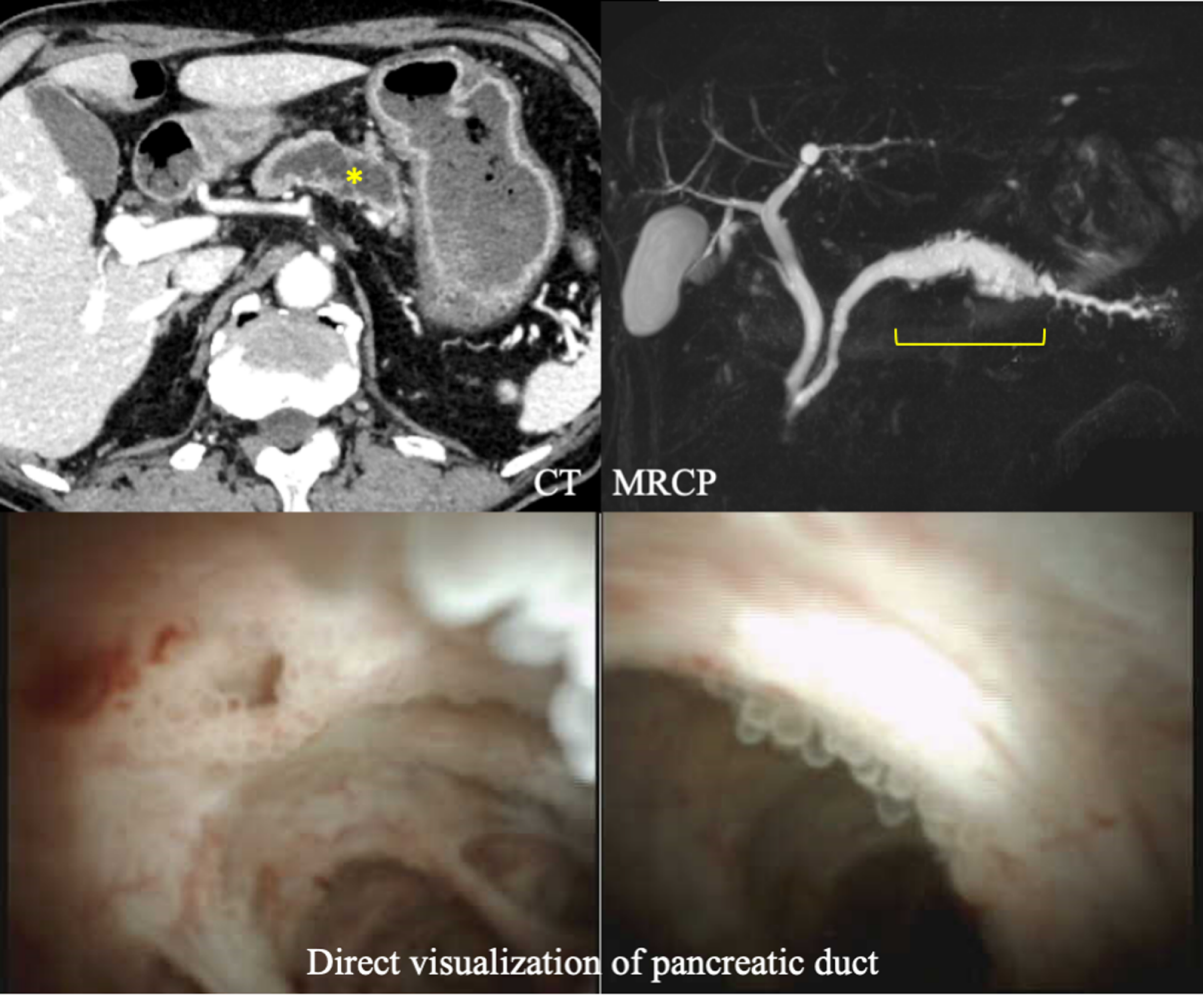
CT and MRCP showed that the main pancreatic duct was dilated to a diameter of 10 mm along a length of 50 mm. No mural nodules or enlarged peripancreatic lymph nodes were observed. The lower pictures show direct visualization of the papillary epithelium in the main pancreatic duct. CT, computed tomography; MRCP, magnetic resonance cholangiopancreatography
